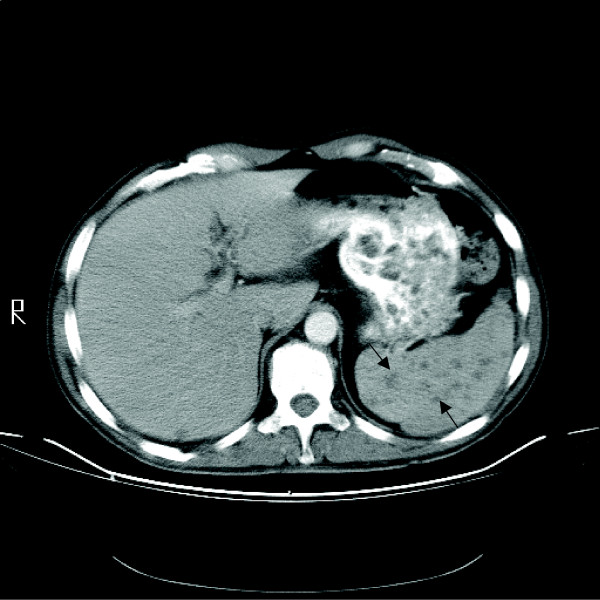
Cross sectional cut of CT scan of abdomen at the time of clinical presentation. Multiple hypoechoic densities are present in the spleen (arrows).
